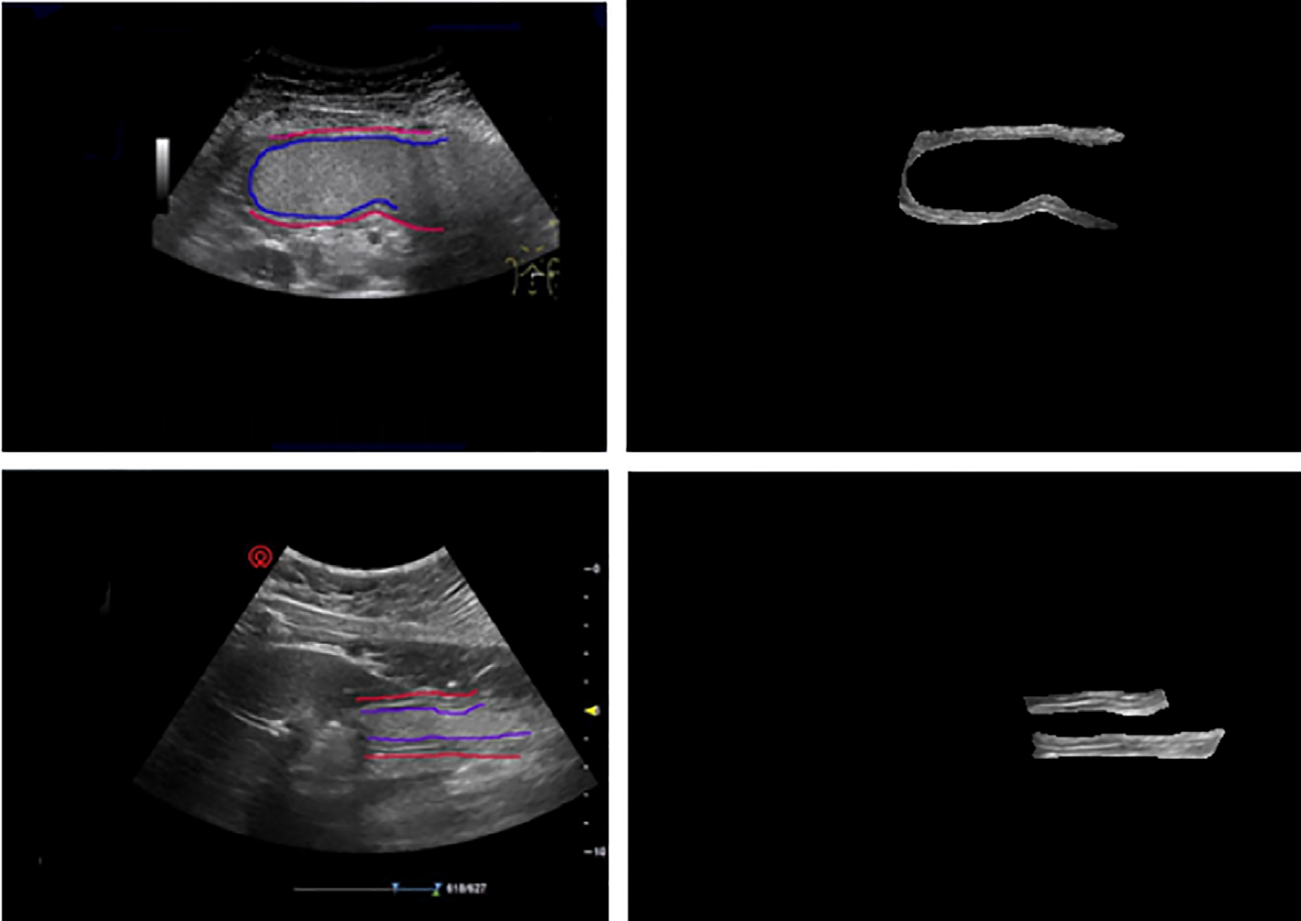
The prediction results of gold standard and our model. After training, the model can accurately predict the gastric wall area in gastric ultrasound images.
